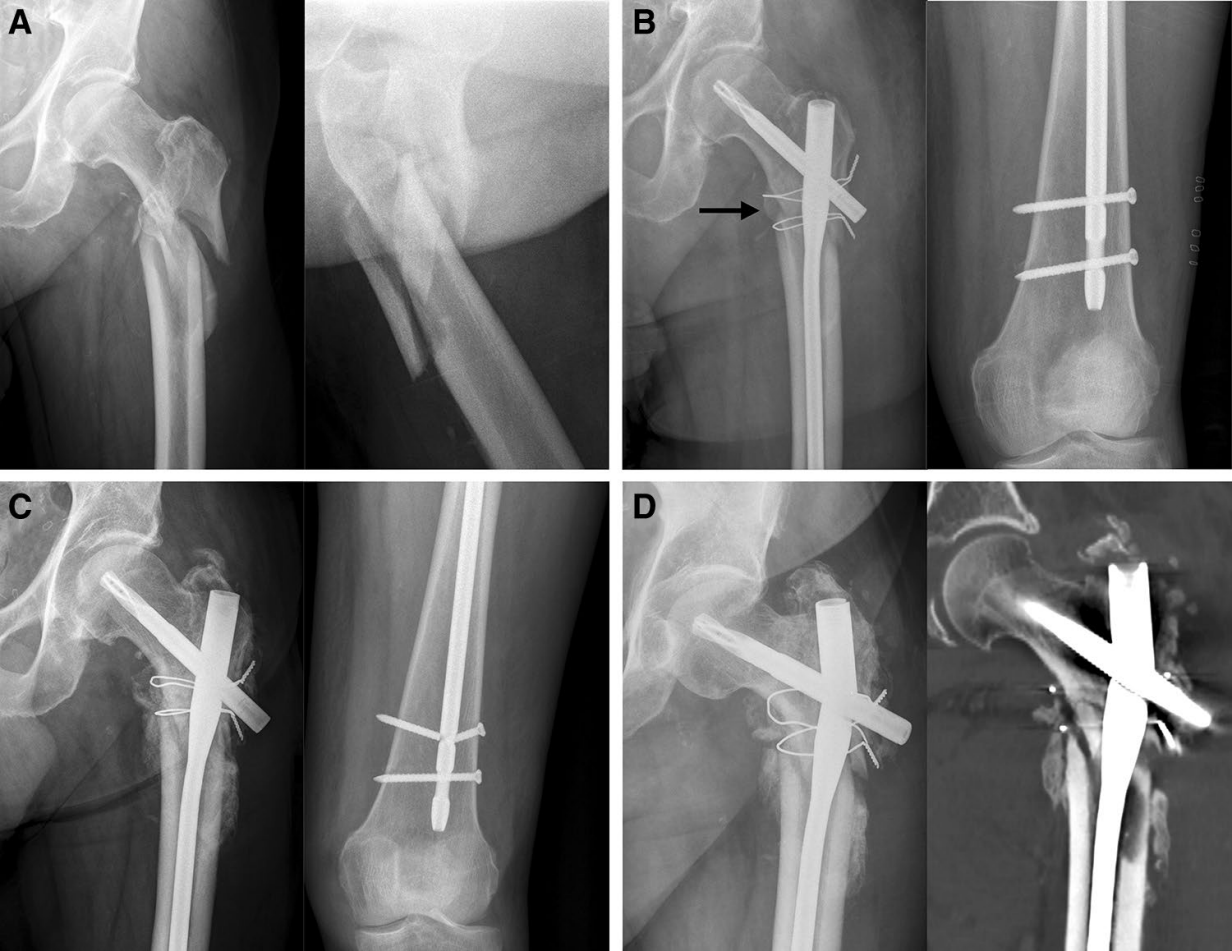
Seventy two-year-old male after a fall at home. a Radiographs obtained at admission showing a Seinsheimer Type IV fracture. b Postoperative radiographs after open reduction, cerclage wiring and intramedullary nailing: varus malalignment (as indicated by the displacement of the proximal medial cortex into the medullary canal with slight varus angulation relative to the distal medial cortex) and lack of medial cortical support due to nonanatomic reduction (black arrow), distal static and dynamic locking. c Unscheduled radiographs after 9 weeks due to persistent pain: no loss of reduction, timely callus formation, breakage of the static locking bolt and autodynamisation of the nail. There was no breakage of the static locking bolt at the previous routine controls. d Unscheduled radiograph (left) and CT scan (right) after 7 months due to suddenly increasing pain: nail breakage and no fracture healing
